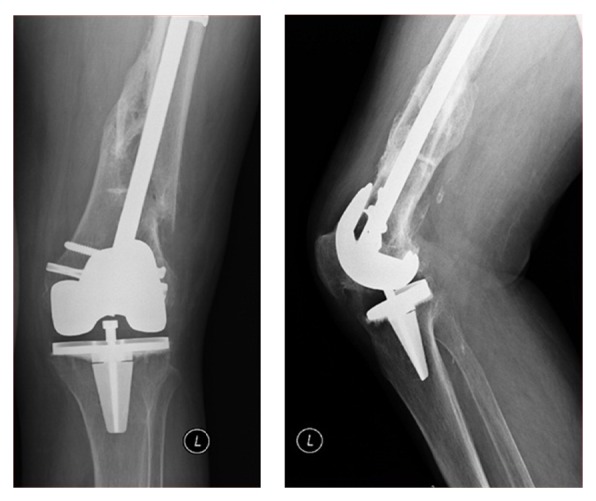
24 months of postoperative AP and lateral left knee X-rays showing complete consolidation of the fracture.
